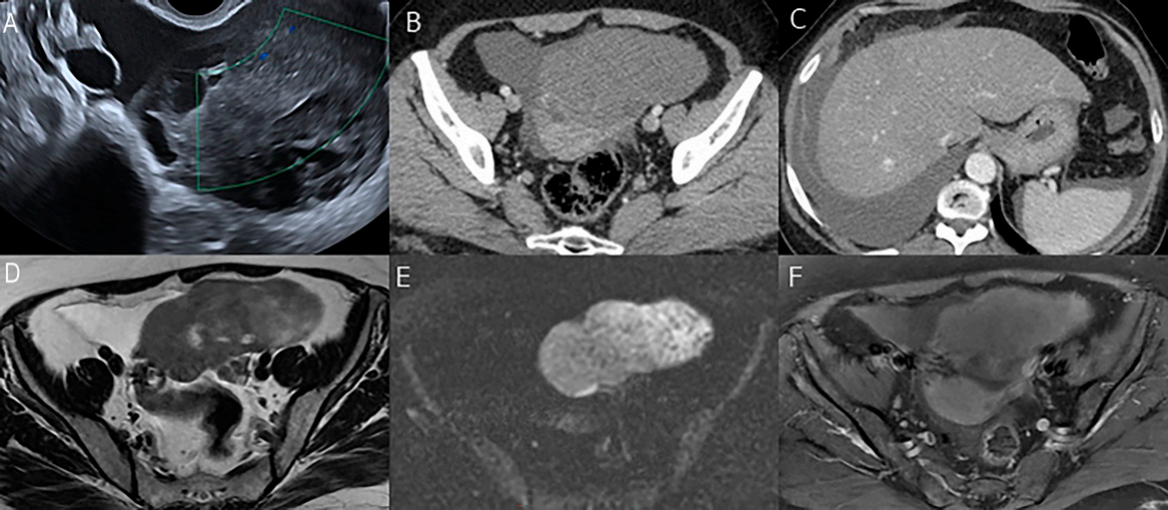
Left ovarian Fibrothecoma in a 55-year-old woman presented with pelvic pain. (A) Color Doppler transvaginal sonography shows an isoechoic lesion with faint vascularity. (B and C) Postcontrast CT axial images show a hypovascular left ovarian lesion with moderate ascites and right-sided pleural effusion (Meigs’ syndrome). (D) Axial T2-WI revealed a lobulated left ovarian solid lesion of predominant intermediate signal with focal slight hyperintensity and minute cystic changes. (E) Axial DWI (high *b* value = 1000) revealed a predominant intermediate signal. (F) Axial fat-suppressed postcontrast T1-WI revealed mild enhancement less than the myometrium.
